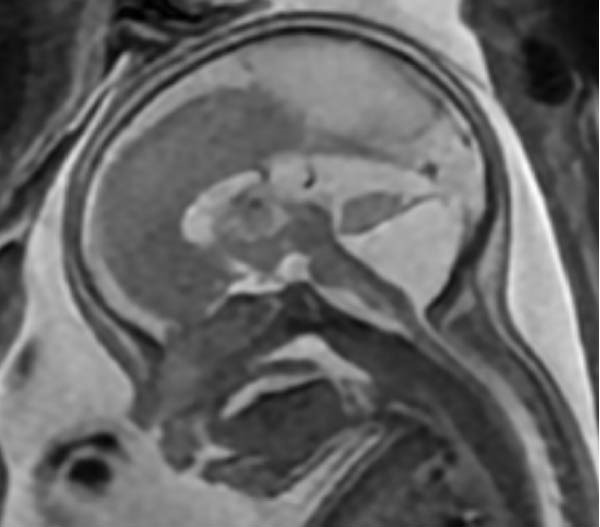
Fetal neuro‐MRI, T2‐sequence, showing a hypoplastic vermis, a broad ponto‐vermian angle, a blunt fastigium recess and a tail sign.
